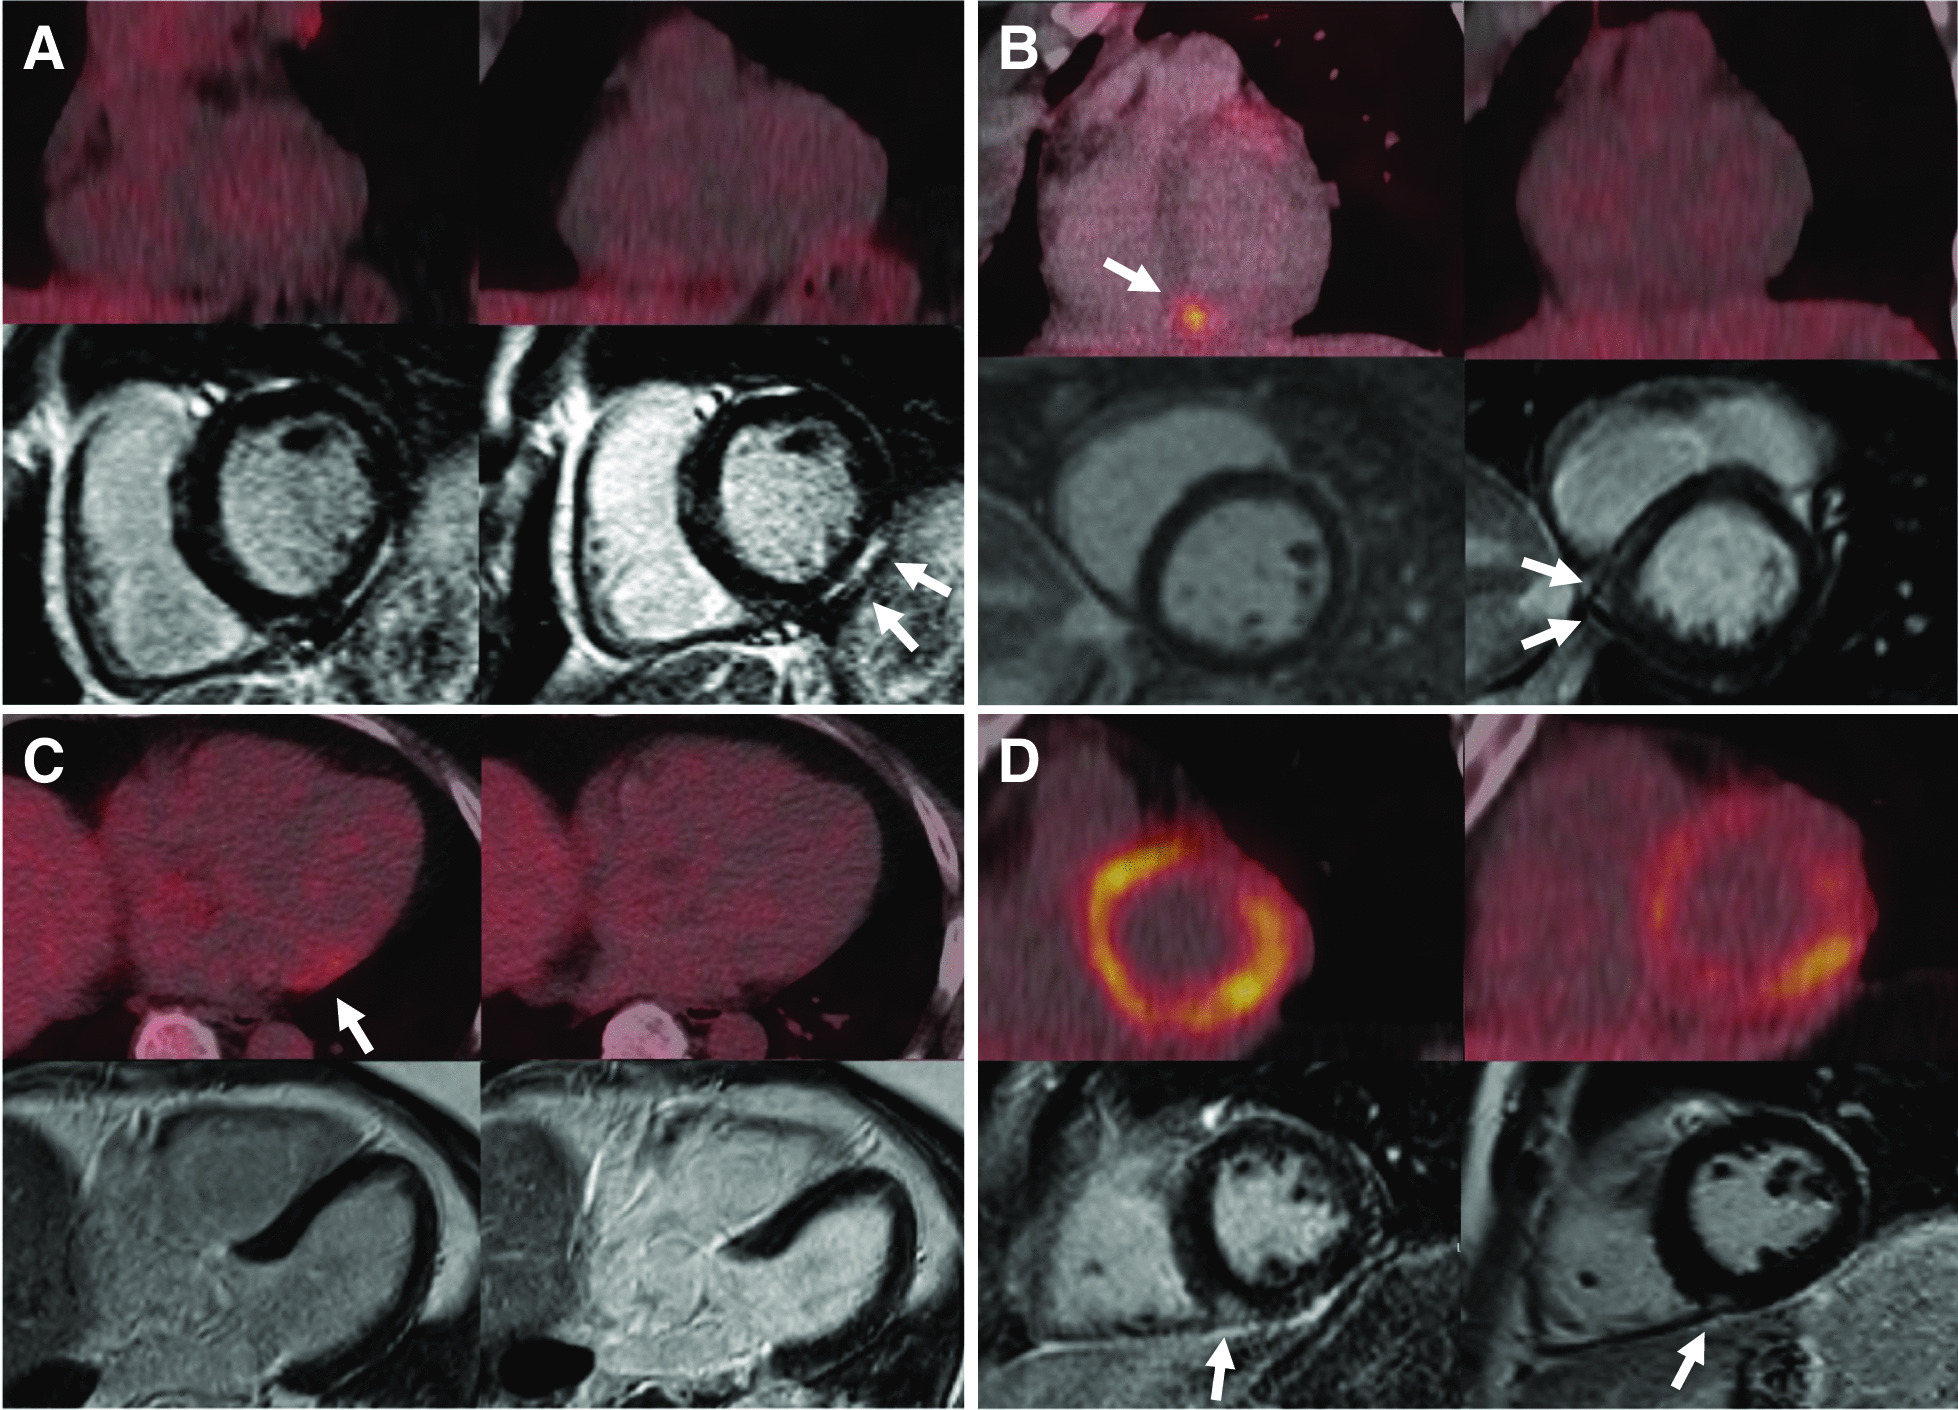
Examples of different FDG PET/CT and CMR patterns. In every image, baseline FDG PET/CT and CMR are shown on the left and repeated imaging on the right. A 48-year-old male patient who showed LGE uptake infero-lateral at first CMR (white arrows, short-axis view) without cardiac FDG-uptake (CMR+/PET−). The LGE increased at 2nd CMR with also increased T2-weighted signal (not shown); however, still no cardiac FDG-uptake was seen (CMR+/PET−), while the patient did not receive any immunosuppressive treatment. He was reclassified as probable CS. B A 36-year-old female patient who showed focal FDG-uptake infero-septal (white arrows) without LGE on CMR at baseline (CMR−/PET+). Between first and 2nd MDT, she was started on methotrexate 15 mg/week due to pulmonary sarcoidosis. Repeated imaging showed complete remission of cardiac FDG-uptake; however, CMR showed new LGE infero-septal (short-axis view, white arrows) and she was classified as CMR+/PET−. This patient was diagnosed with probable CS. C A 56-year-old male with focal FDG-uptake in the antero-lateral wall (white arrow, SUVmax 4.3) at baseline. He showed no LGE uptake on CMR (4 chamber view) and was classified as CMR−/PET+. The FDG-uptake was suspected to be physiologic and repeated imaging showed no cardiac FDG-uptake or LGE on CMR (CMR−/PET−). This patient received no immunosuppressive treatment between both MDT’s and CS was deemed “unlikely”. D A 47-year-old male patient who showed initial LGE inferoseptal on CMR (white arrow, short-axis view). However, after repeated imaging this LGE was interpreted as inferior hinge point fibrosis and not suspect for CS. Both FDG PET/CTs showed diffuse cardiac FDG-uptake (CMR+/PET+, CMR−/PET+). This patient did not receive any immunosuppressive therapies and was reclassified as “unlikely” CS
